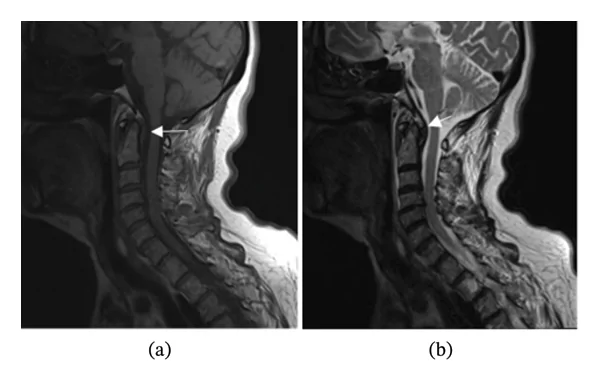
MRI demonstrating calcification along the transverse ligament of the odontoid process. (a) T1 weighted. (b) T2 weighted.
